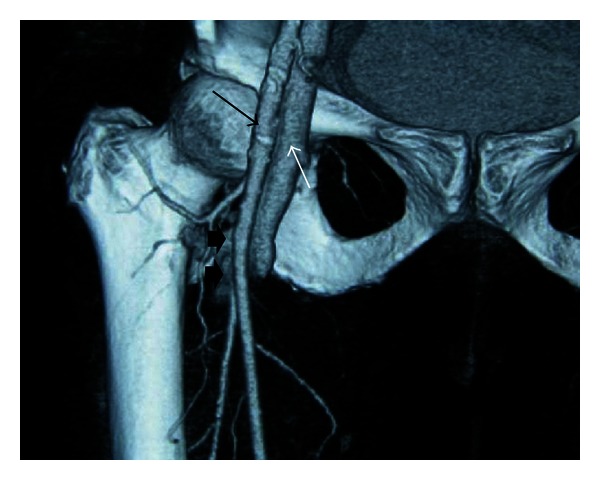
CT angiography examination of the patient. Contrast passage between the femoral artery and vein (arrow heads) is demonstrated. (Black arrow: common femoral artery, white arrow: common femoral vein).
